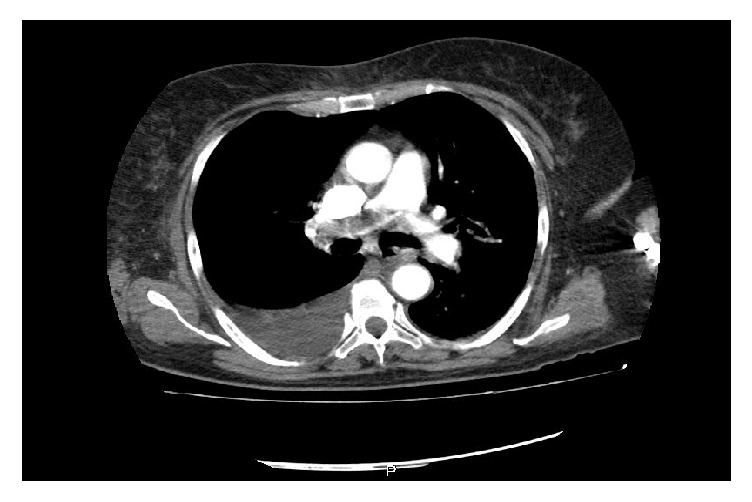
CT pulmonary angiography showing a large defect in the filling of the bifurcation and the right pulmonary artery.
